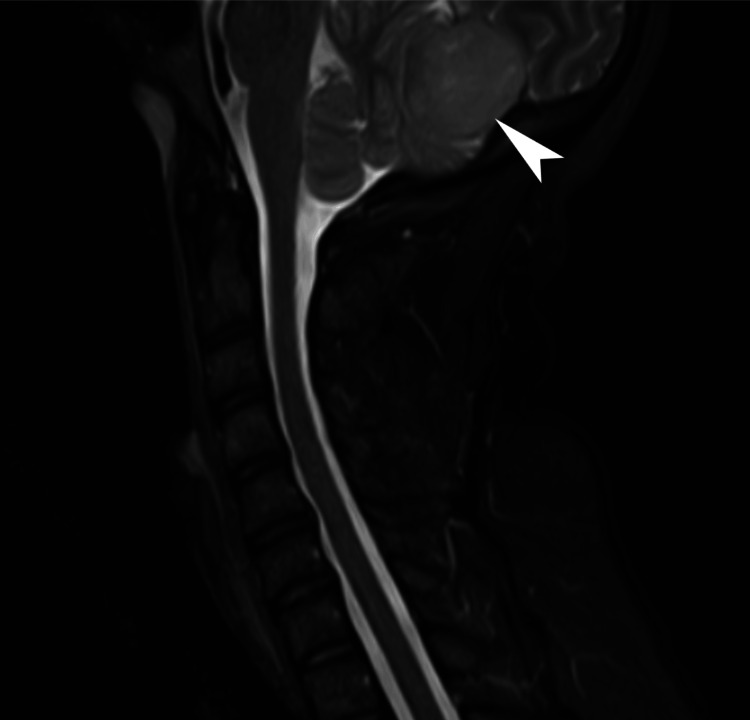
Magnetic resonance imaging of the cervical spine. This mid-sagittal short tau inversion recovery sequence demonstrates an unremarkable cervical spine; however, there is a 3.2 cm posterior fossa mass (arrowhead).
